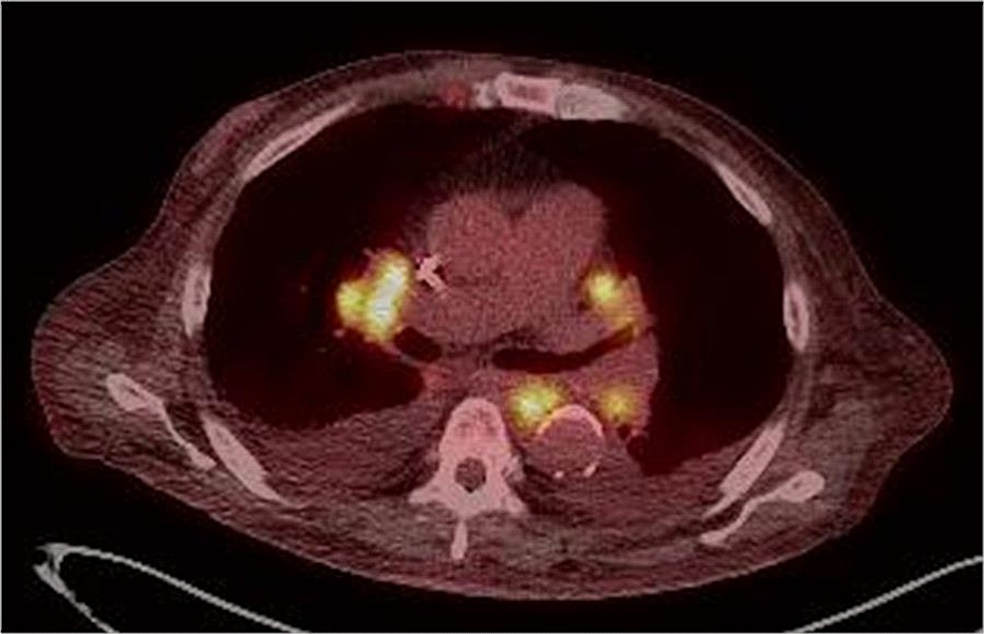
The yellow highlighted regions on this patient's PET/CT represent areas of lymphadenopathy concerning for malignancy PET/CT: positron emissions tomography/computed tomography
